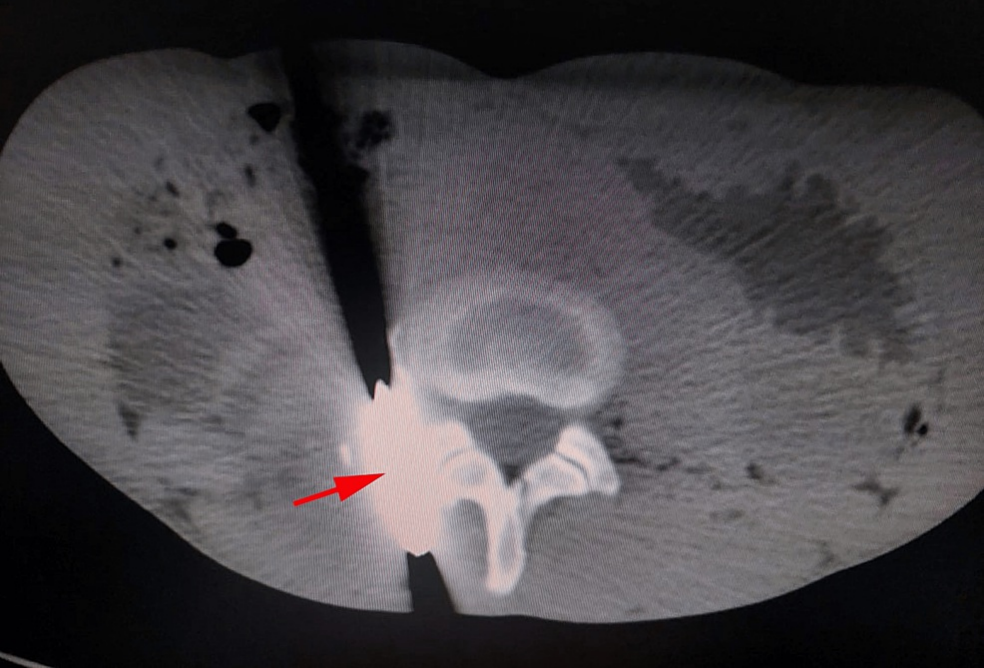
Abdominal computed tomography scan The image reveals the presence of a 52 x 20 mm metallic foreign body in the lumbar region at the level of L3 and L4 vertebral bodies. Exploration of the left kidney and ureteral duct revealed no anomalies.
